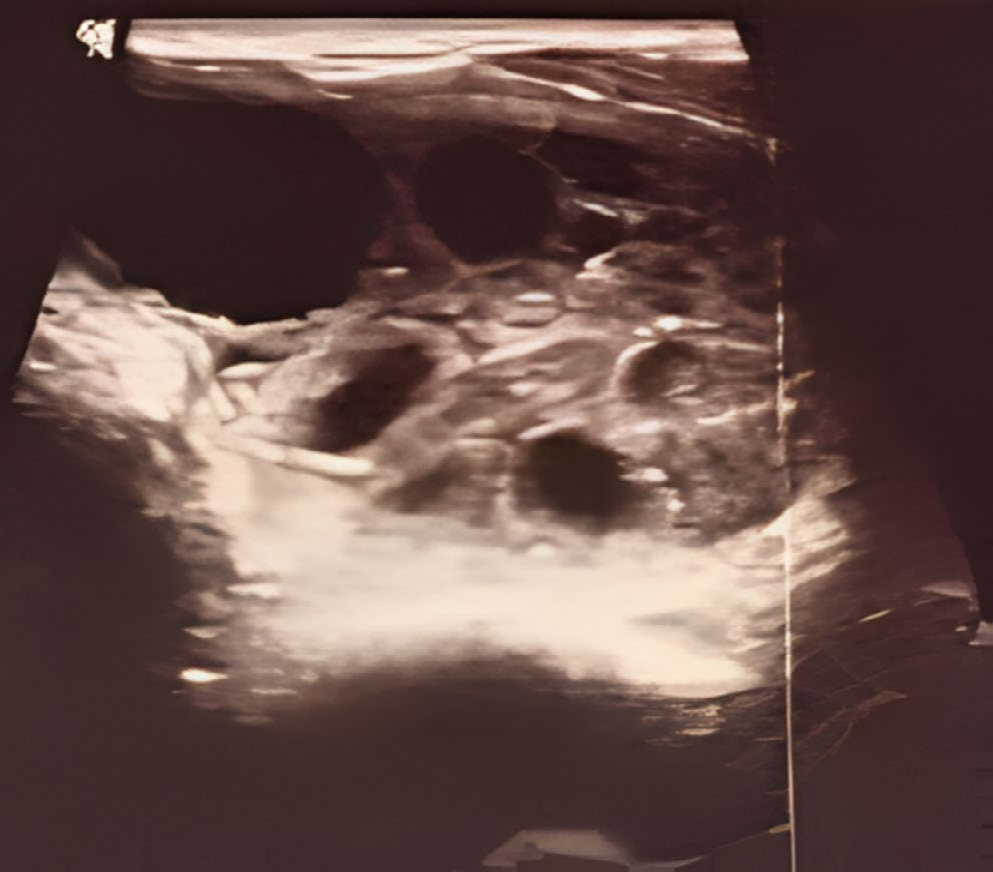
Multiloculated anechoic lesion on sonography.
